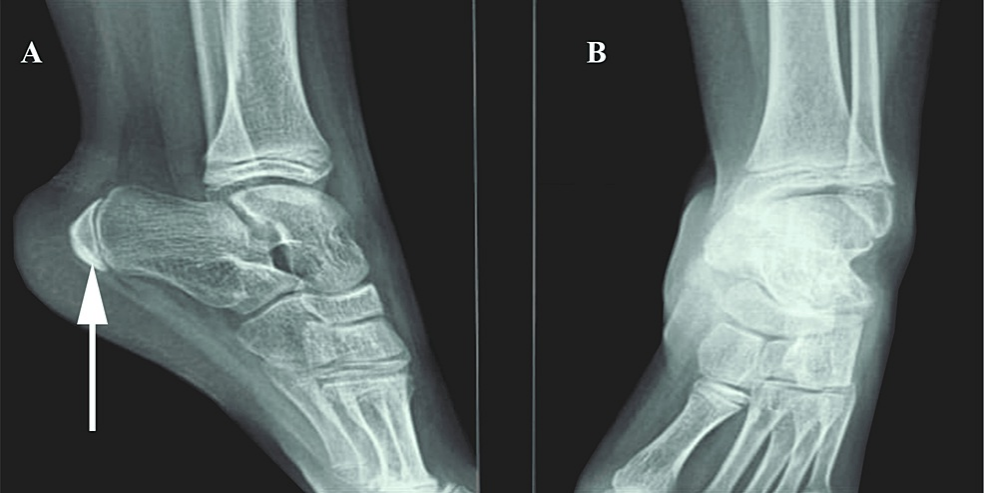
Anteroposterior and lateral radiographs of the left foot demonstrating the presence of calcaneal apophyseal fragmentation (white arrow) associated with increased bone density (A) Lateral X-ray; (B) Posteroanterior X-ray
